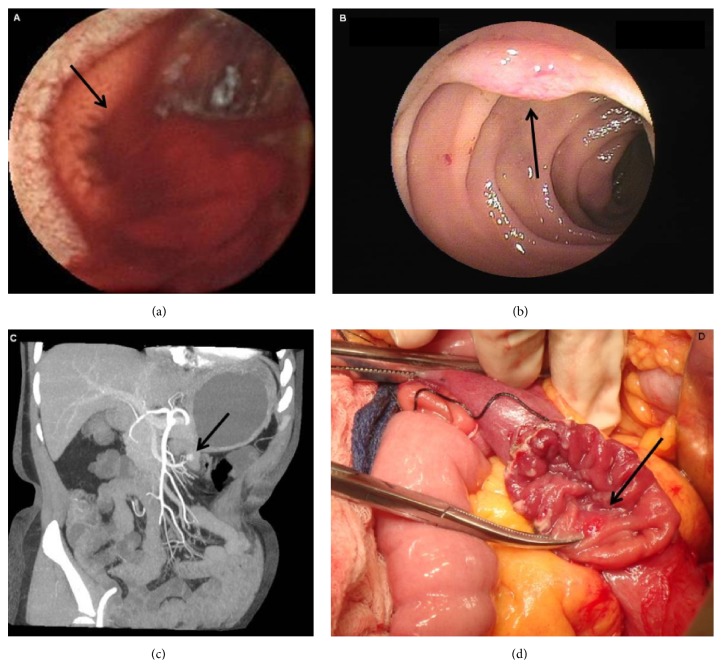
Images in a patient presenting with melena and diagnosed with hemangioma. (a) Image from capsule endoscopy showed active bleeding (arrow) in the proximal jejunum, without evidence of the source of bleeding. (b) Image from double-balloon enteroscopy indicated protruding lesion (arrow) with superficial vascular dilatation in the proximal jejunum. (c) Dual-phase computed tomographic enterography showed a lesion (arrow) in the jejunum with striking enhancement in the arterial phase. (d) Macroscopic appearance of the dissected segment of jejunum with the lesion (arrow).
